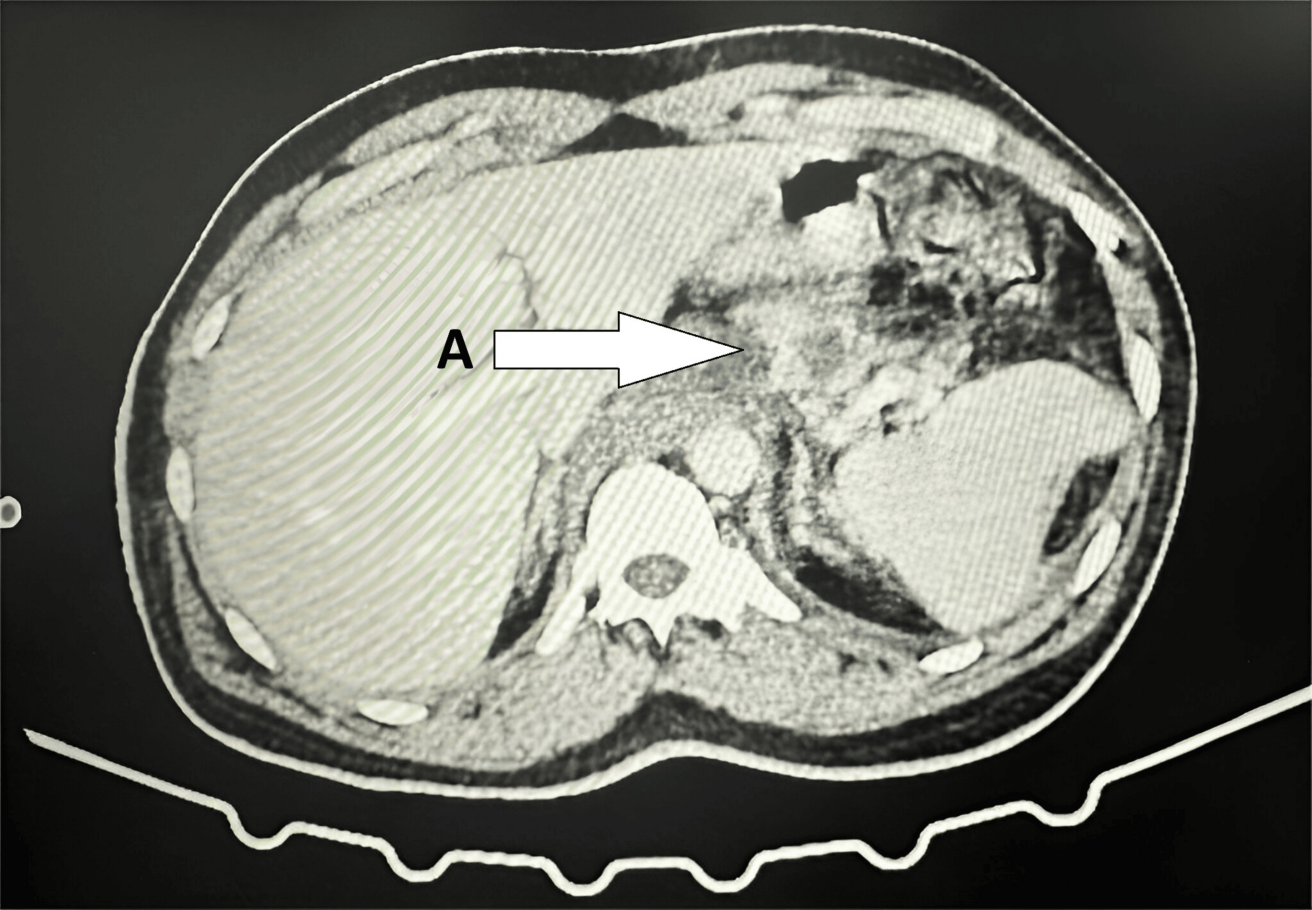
CECT abdomen showing chronic pancreatitis with mediastinal extension of a pseudocyst, peripancreatic fluid collections, and possible ductal disruption in the tail region. A: Chronic pancreatitis with mediastinal extension of a pseudocyst, peripancreatic fluid collections, and possible duct disruption in the tail region. CECT, contrast-enhanced computed tomography
